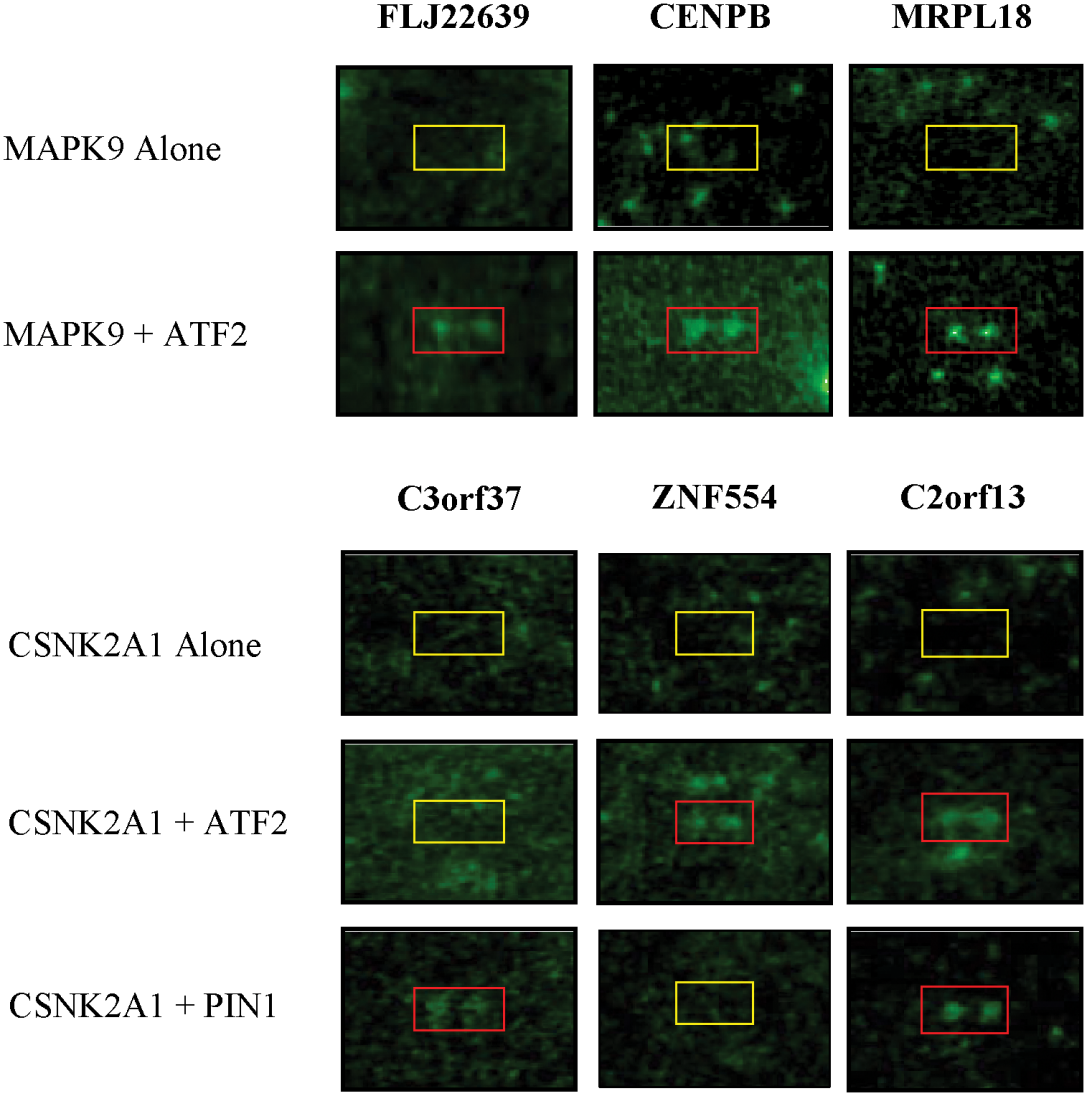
Experimental validations for *CSNK2A1* and *MAPK9*. A human proteome microarray, comprised of 17,000 individually purified human proteins in full-length, was used to perform phosphorylation reactions with CKII (CSNK2A1) and JNK2 (MAPK9) in the presence or absence of their predicted scaffold proteins, ATF2 and PIN1. Phosphorylation signals were detected by exposure of the human proteome microarrays to X-ray film. Positive hits in red boxes were identified by visual inspection.
